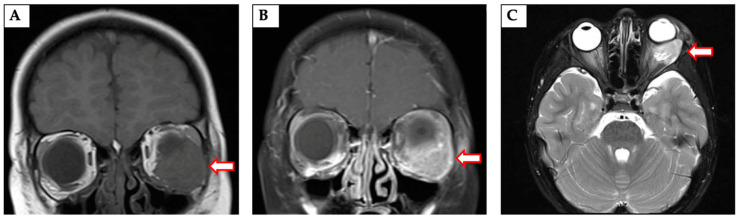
Magnetic resonance imaging (MRI) of an orbital primary tumor (arrows) in coronal T1 pre-contrast (Panel A) and post-contrast (Panel B) and axial T2 (Panel C) sequences. Panel (B) demonstrates the utility of contrast administration and fat suppression in delineating tumor for diagnosis and treatment planning.
